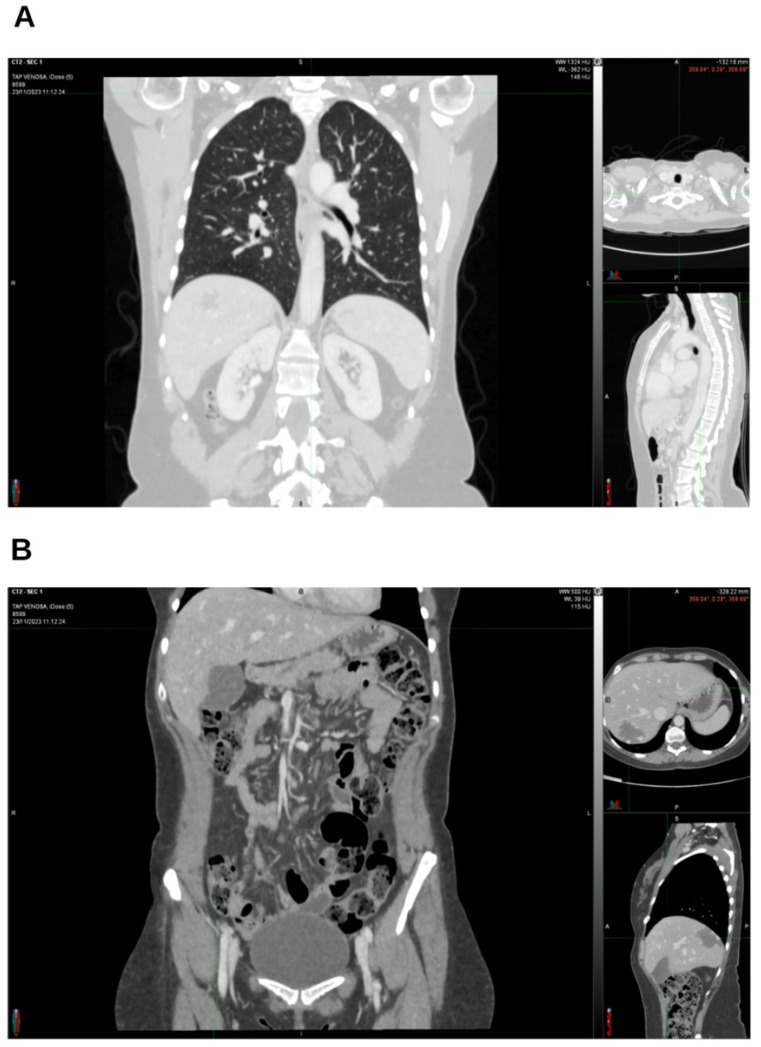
In November 2023, the lung (A), abdomen, and pelvis (B) CT scan was still negative.
